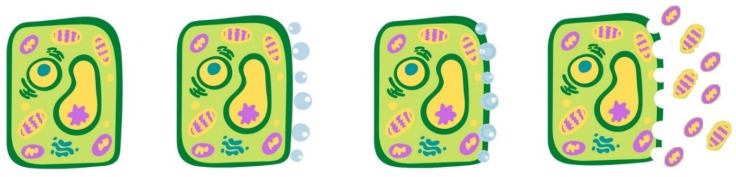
Mechanism of cell rupture induced by ultrasounds: cavitation bubble generation, bubble collapse, and cell wall fragmentation.
